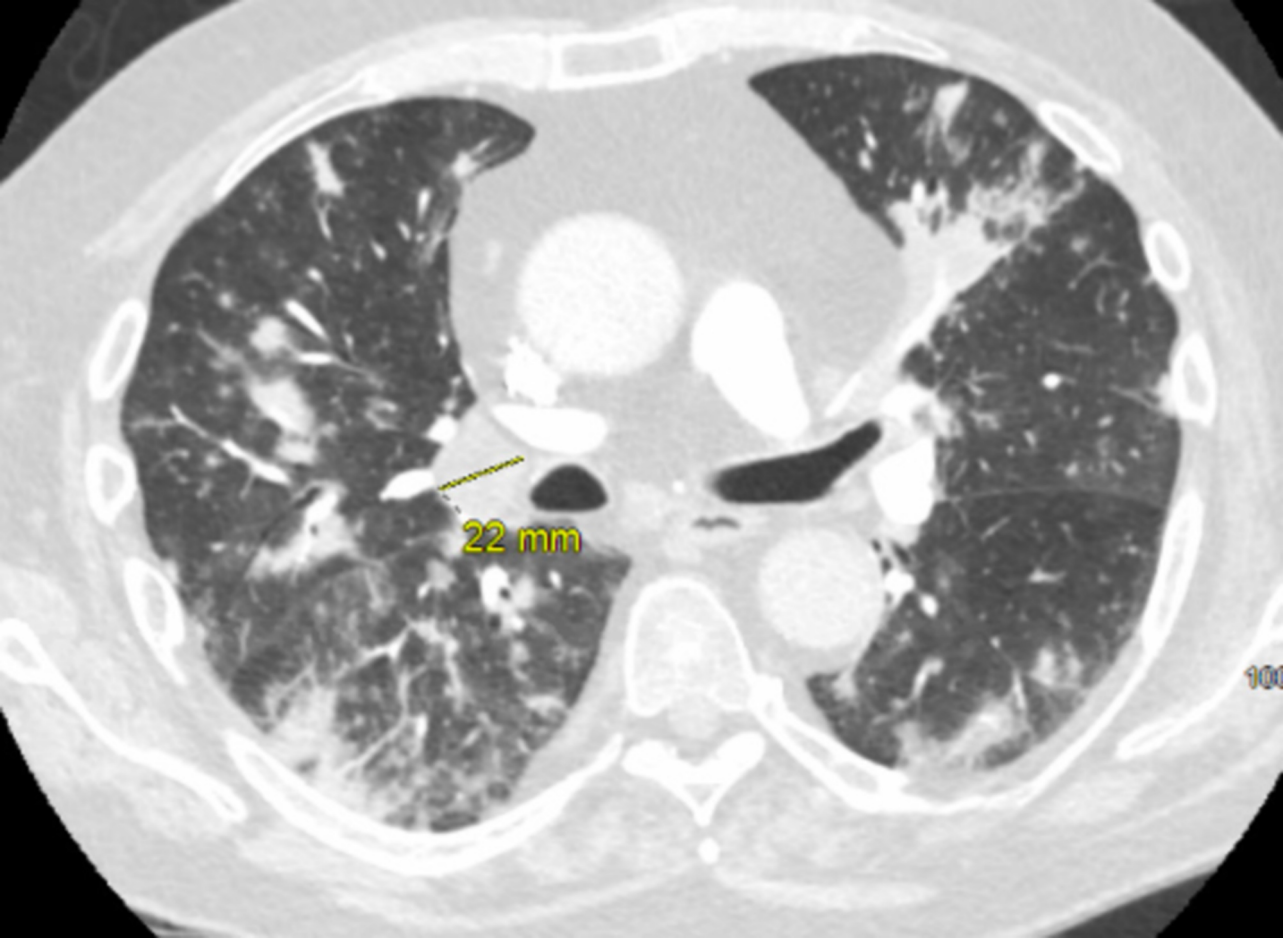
CT angiogram chest showing multifocal infiltrates and prominent right hilar lymphadenopathy.
